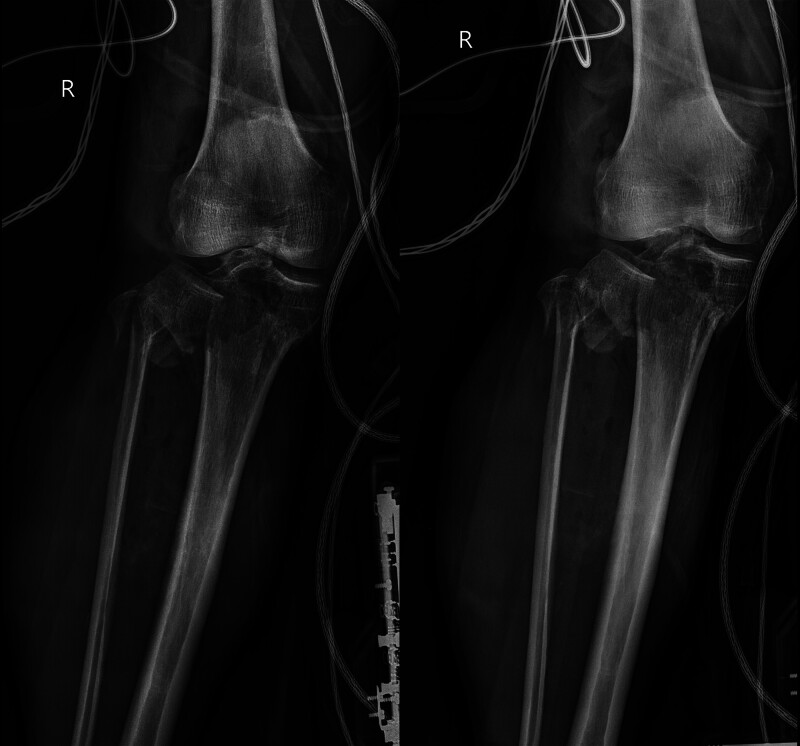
Radiographs showed a tibial plateau comminuted fracture of Schatzker type V (Fig. 1; date of examination: August 3, 2021). (A) Plain x-ray of the knee with anteroposterior view. (B) Plain x-ray of the knee with lateral view.
